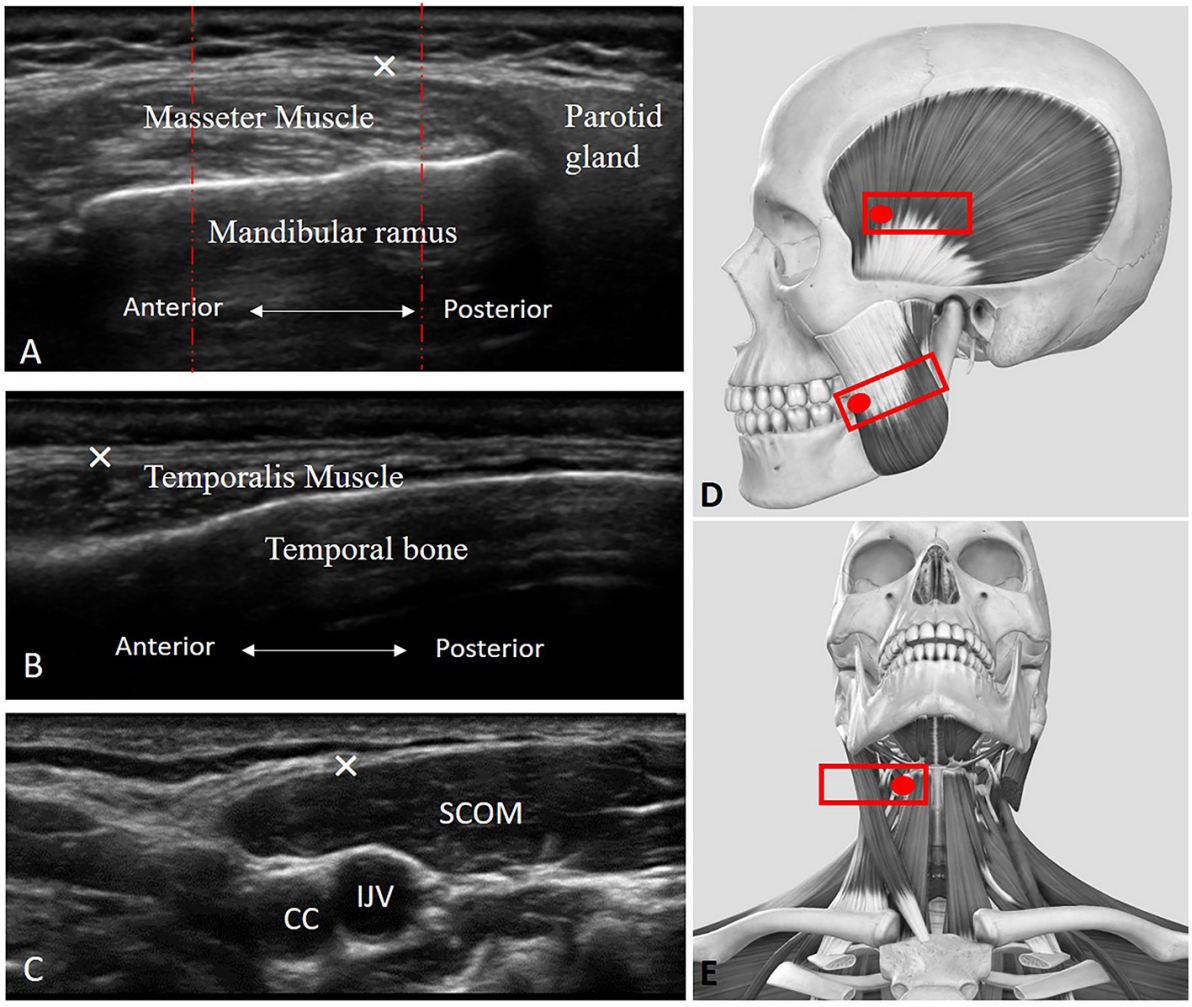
Ultrasound (US) images of the masseter (A), temporalis (B), and scom (C) muscles with their fasciae. CC, common carotid artery; IJV, internal jugular vein; SCOM, sternocleidomastoid muscle; ×, deep fasciae. (D) Transducer placement for scanning of the masseter and temporalis muscles. (E) Transducer placement for scanning of the SCOM muscle. Red dashed lines: show how the three measures were taken in the three equidistant regions per image/structure identified.
